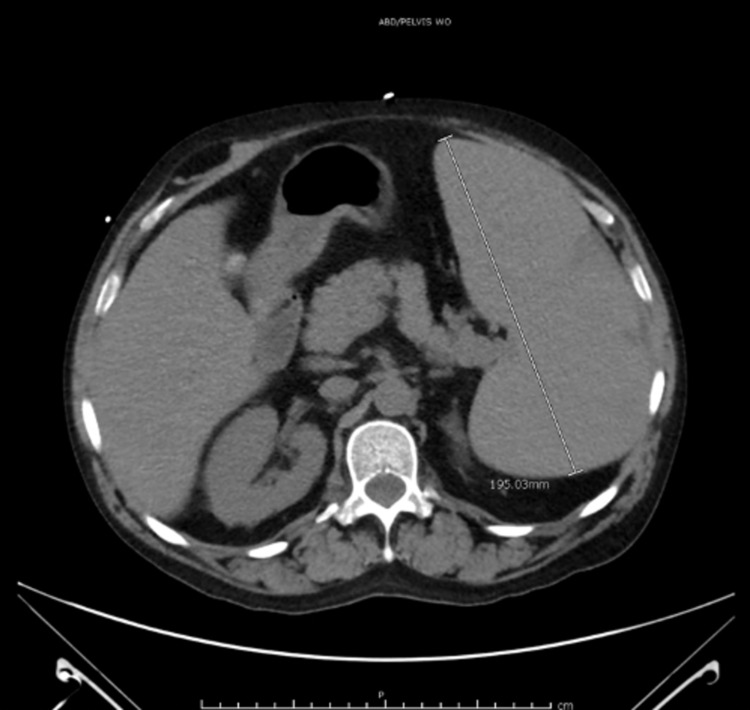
CT of the abdomen showing splenomegaly up to 19.5 cm with hypodensities at the periphery consistent with splenic infarcts.
